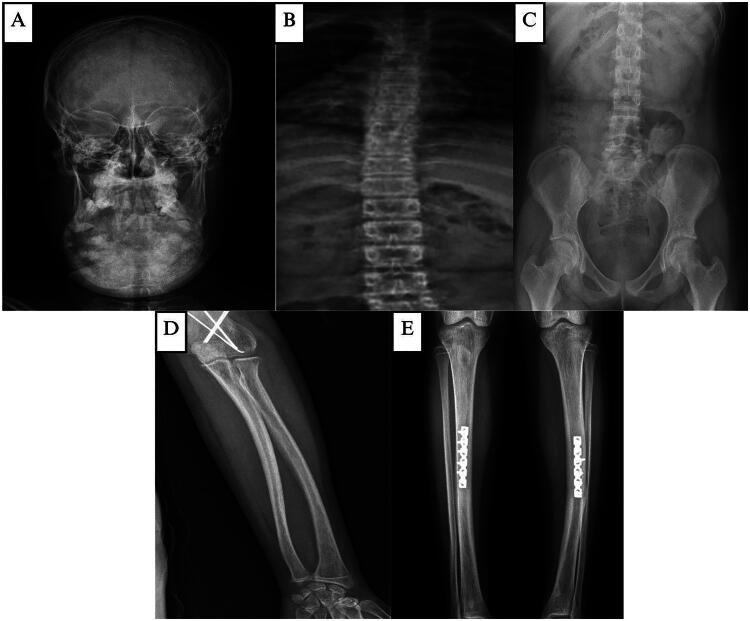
(A) Skeletal survey of the fourth patient, X-ray of the head shows good bone trabeculation, inhomogeneous opacity in the mandibular region, amorphous shape, clear borders with regular edges, accompanied by calcification. (B–E) X-ray of the vertebrae, pelvic, upper and lower extremity shows good bone structure and trabeculation. No osteolytic or osteosclerotic bone are visible.
